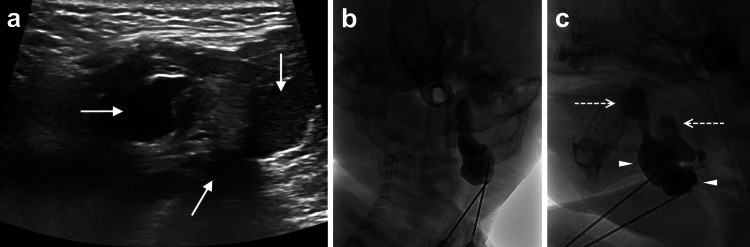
(a) Ultrasound showed three left submandibular lesions (solid arrows), with one lesion displaying increased, heterogenous internal echogenicity compared to two other more hypoechoic lesions. Following aspiration of the lesions, a cystogram, on coronal (b) and sagittal (c) views was performed by injecting fluoroscopic contrast into two of the sites, which demonstrated discrete fluid pockets (arrowheads) with discrete extensions from the left sublingual space (dashed arrows).
